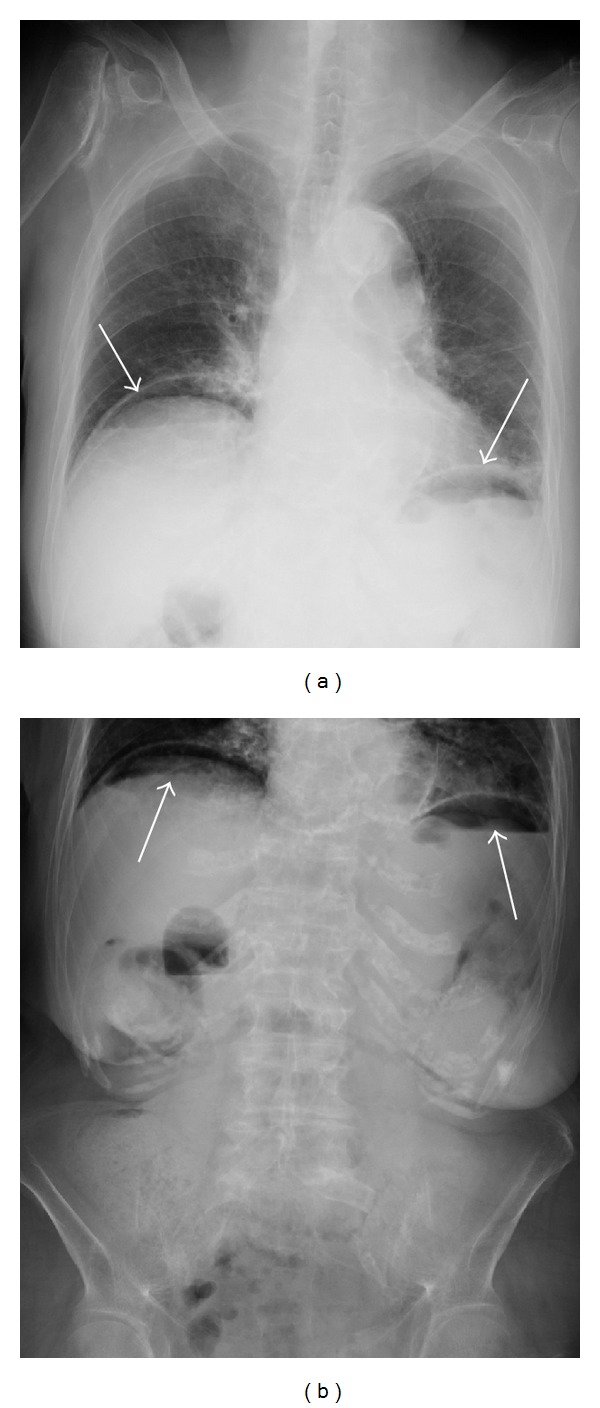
Chest (a) and abdominal (b) radiography revealed free air under bilateral diaphragms.
